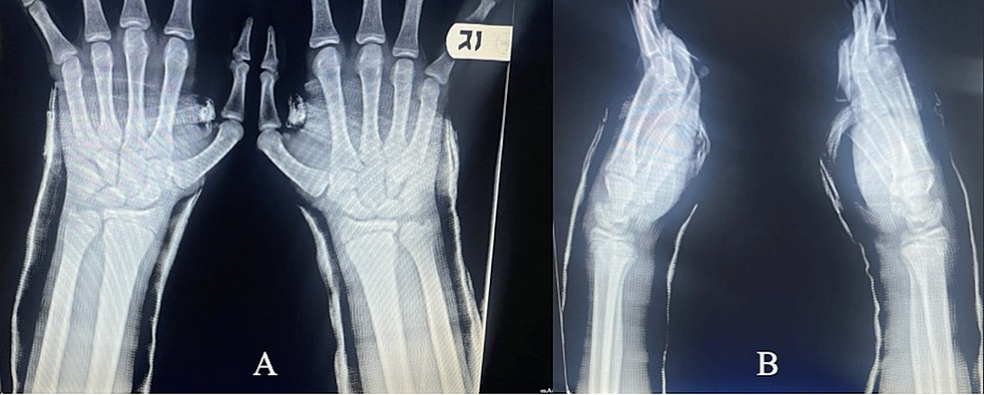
Bilateral wrist radiographs five and a half weeks post-injury Posteroanterior (A) and lateral (B) radiographs of the left and right wrists immediately prior to short arm cast removal at five and half weeks post-injury demonstrating interval healing of the bilateral distal radii fractures
